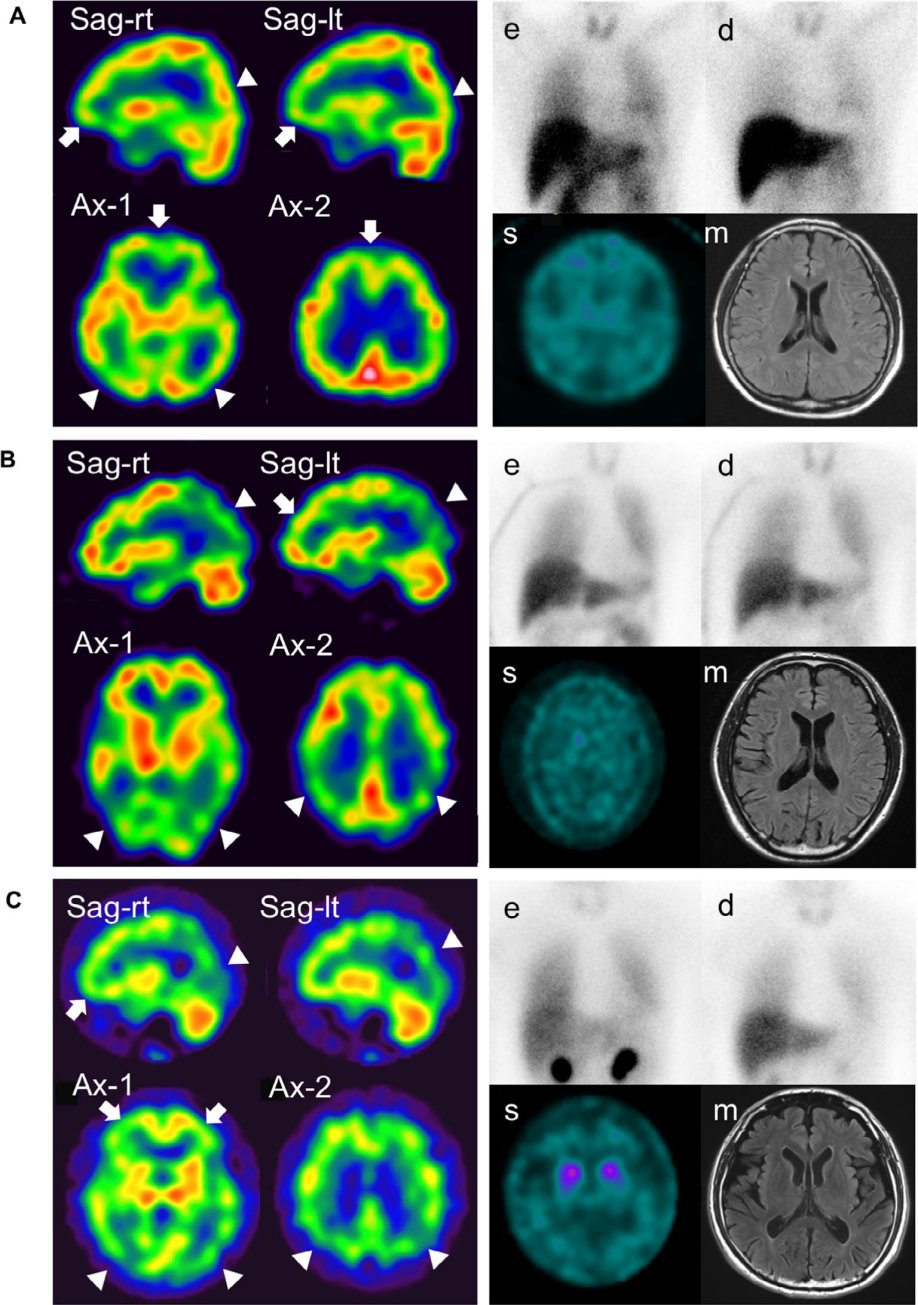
Clinical images of carriers with *SNCA* p.V15A. (A) Family A subject III‐10. (B) Family A subject III‐11. (C) Family B subject III‐1. Left: single‐photon emission computed tomography (SPECT) with N‐isopropyl‐p‐[^123^I]‐iodoamphetamine (IMP‐SPECT); upper right: ^123^iodine‐metaiodobenzylguanidine myocardial scintigraphy at early (e) and delay (d) phases; lower right: SPECT imaging with ^123^I‐ioflupane (s) and brain magnetic resonance imaging of fluid‐attenuated inversion recovery (m). Arrows and arrowheads indicate decreased perfusion in the frontal lobe and in the occipital lobe, respectively. Images of Ax‐1 and Ax‐2 contain the basal ganglia and the body of the lateral ventricle, respectively. Ax, axial; lt, left; rt, right; Sag, sagittal. [Color figure can be viewed at wileyonlinelibrary.com]
